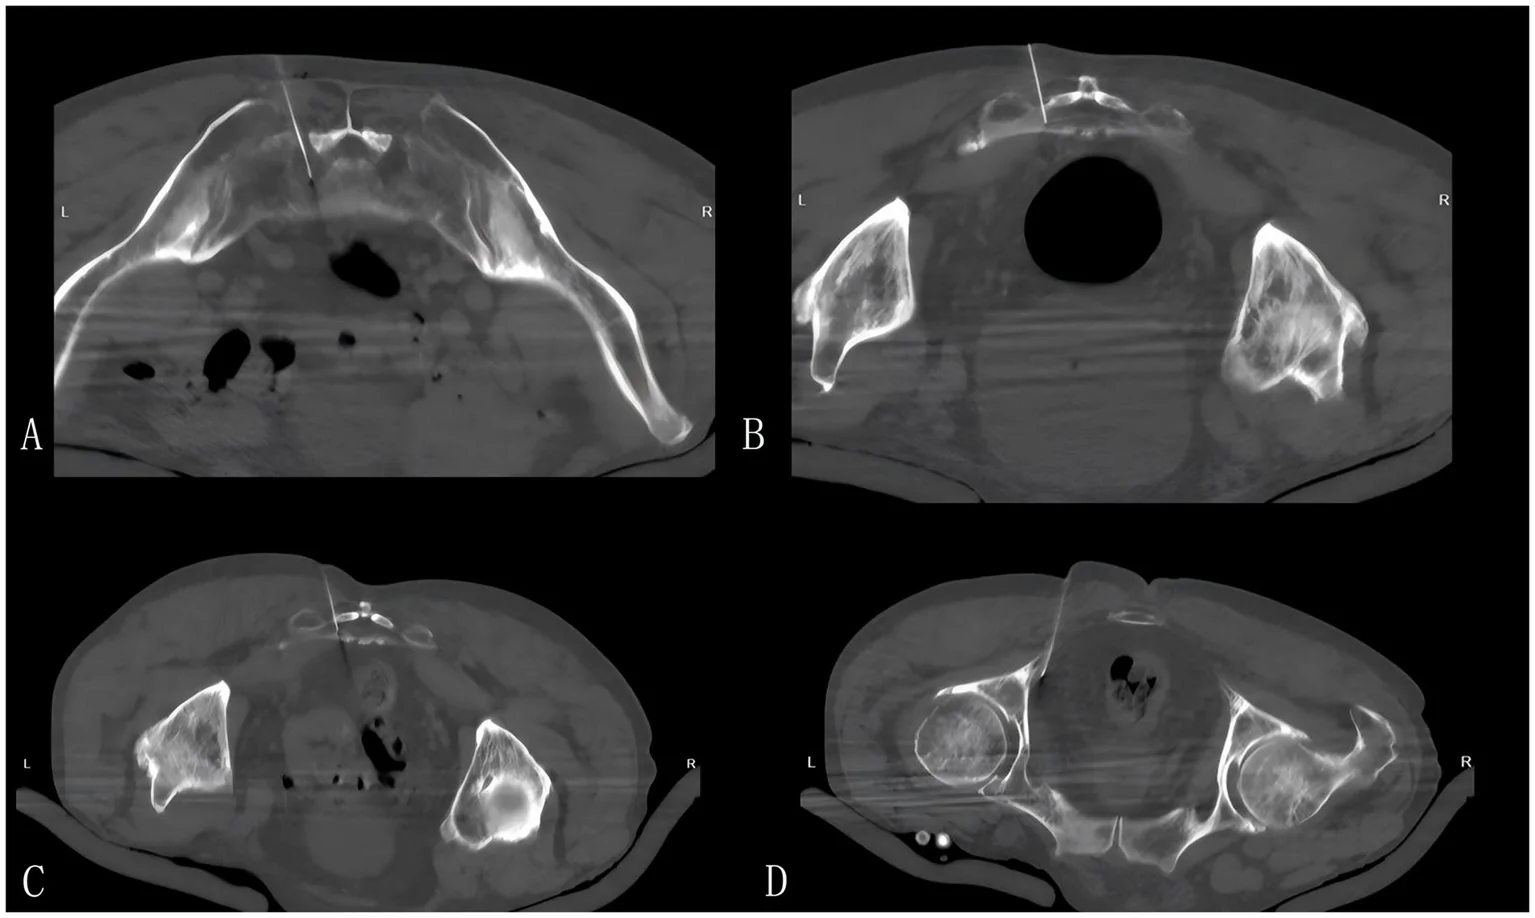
CT-guided needle placement for pulsed radiofrequency (PRF). (A) S2 sacral nerve; (B) S3 sacral nerve; (C) S4 sacral nerve; (D) pudendal nerve in the pudendal canal.
